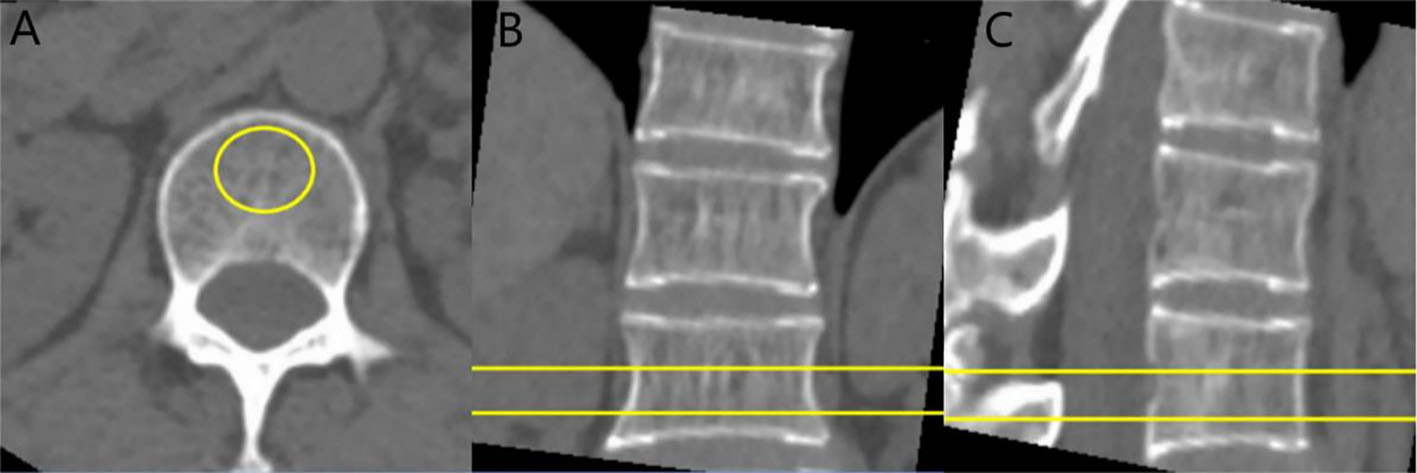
CT value and BMD measurement of vertebral body: cases report. (A) axial image, (B) sagittal image, and (C) coronal image. The ROI is placed on the vertebral cancellous bone, avoiding the vertebral cortical bone and the posterior venous plexus.
